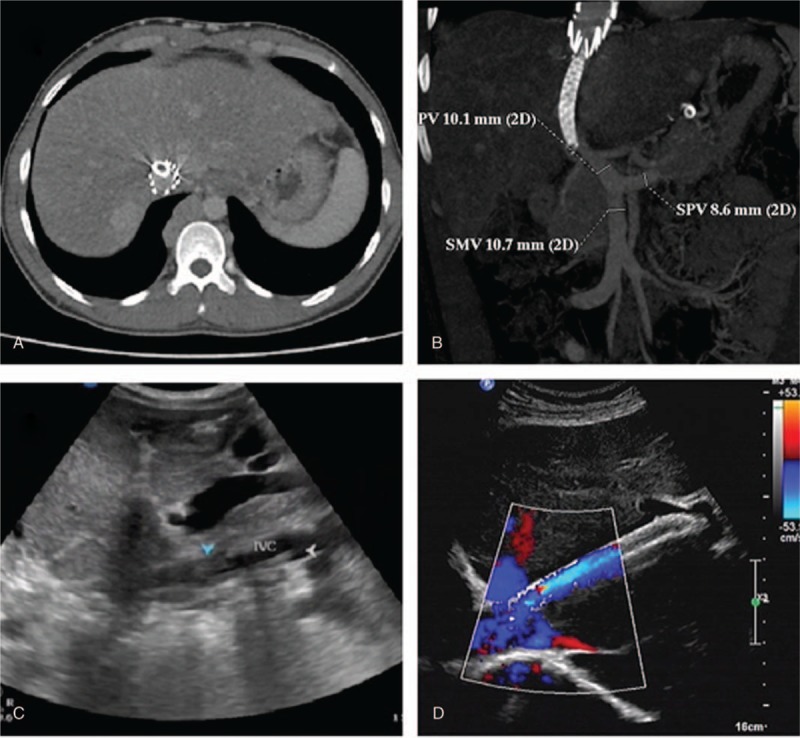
CTA and color Doppler ultrasonograpy test after procedure. The stent shunt and stent in IVC were shown by CTA in coronal (a) and sagittal position (b). The color Doppler ultrasonograpy was used to exam the patency of IVC (c) and stent shunt (d) during follow up. CTA = computerized tomography angiography, IVC = inferior vena cava.
